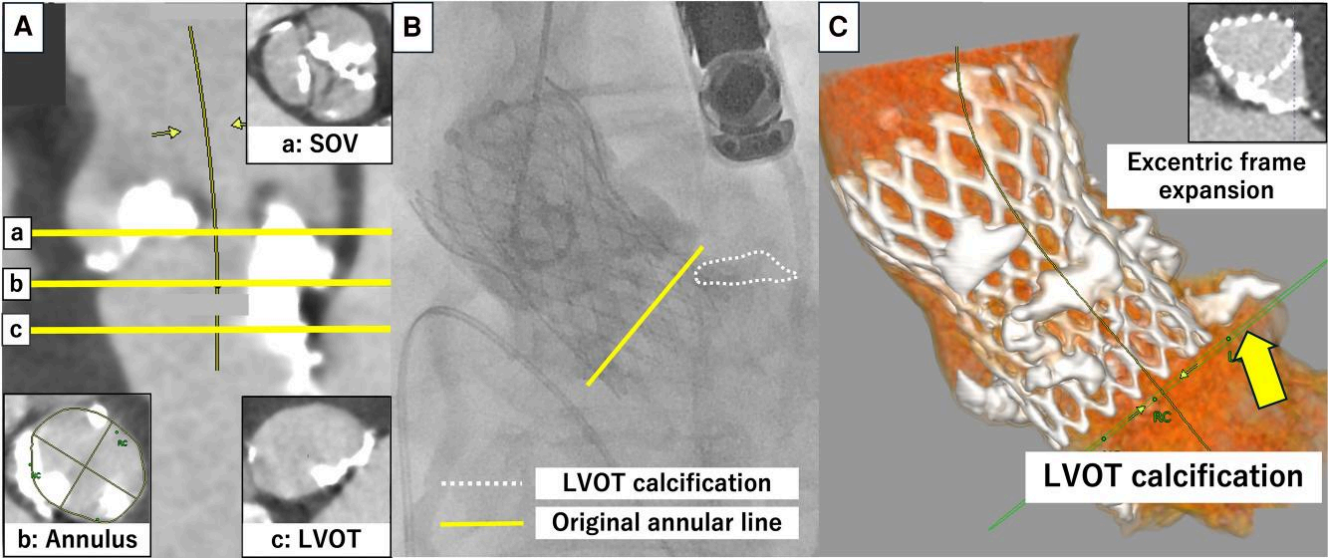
Pre- and post-procedural images of Case 4. (*A*) Pre-procedural computed tomography: a, sinus of Valsalva level; b, annular level; c, left ventricular outflow tract level. (*B*) Aortography after transcatheter aortic valve replacement. (*C*) Post-procedural computed tomography.
